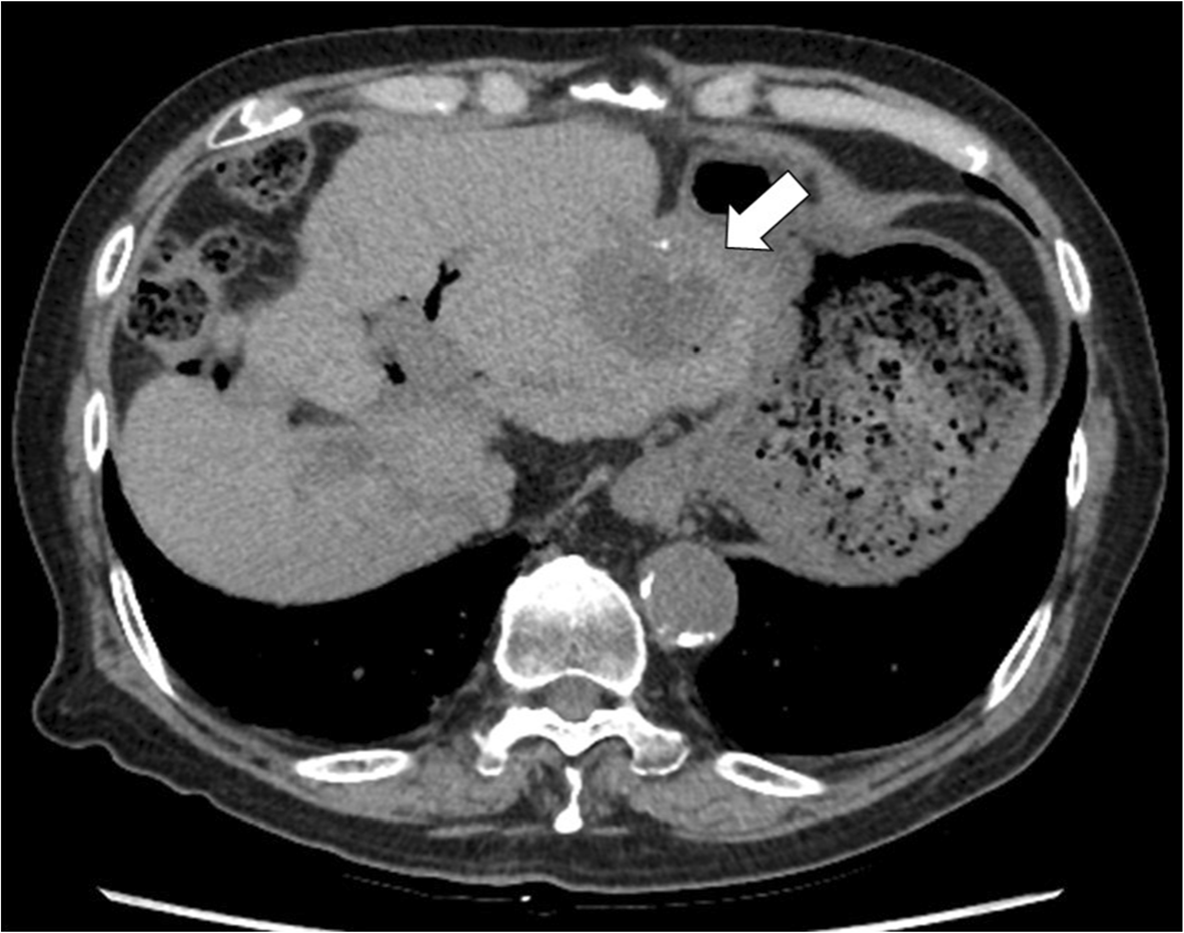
Computed tomography images in the axial plane. A low-density nodule (*white arrow*) in the left lobe is shown. Dynamic computed tomography was difficult to perform in this patient because of decreased renal function
